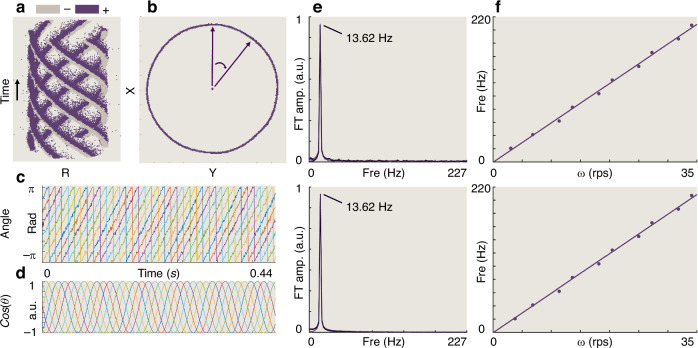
Experimental validation of two singularity-based rotation speed sensing methods. a Time-series polarity data of rotating singularities. R represents the (x, y) plane. b XY distribution of singularity positions with calculated rotation center. c, d Angle extraction for six off-axis singularities (clockwise defined as positive): c Time-series of angle derived from polarity event; d Time-series of cosine angles between singularity positions and geometric center. The individually calculated values for six singularities are represented by six distinct colors. e Fourier spectra calculated from both time-series in c and d (top: polarity-derived angles in c; bottom: position cosine angles in d; same for f). f Linear relationship between measured rotation speed (*x* axis) and preset Doppler frequency shifts (*y* axis)
